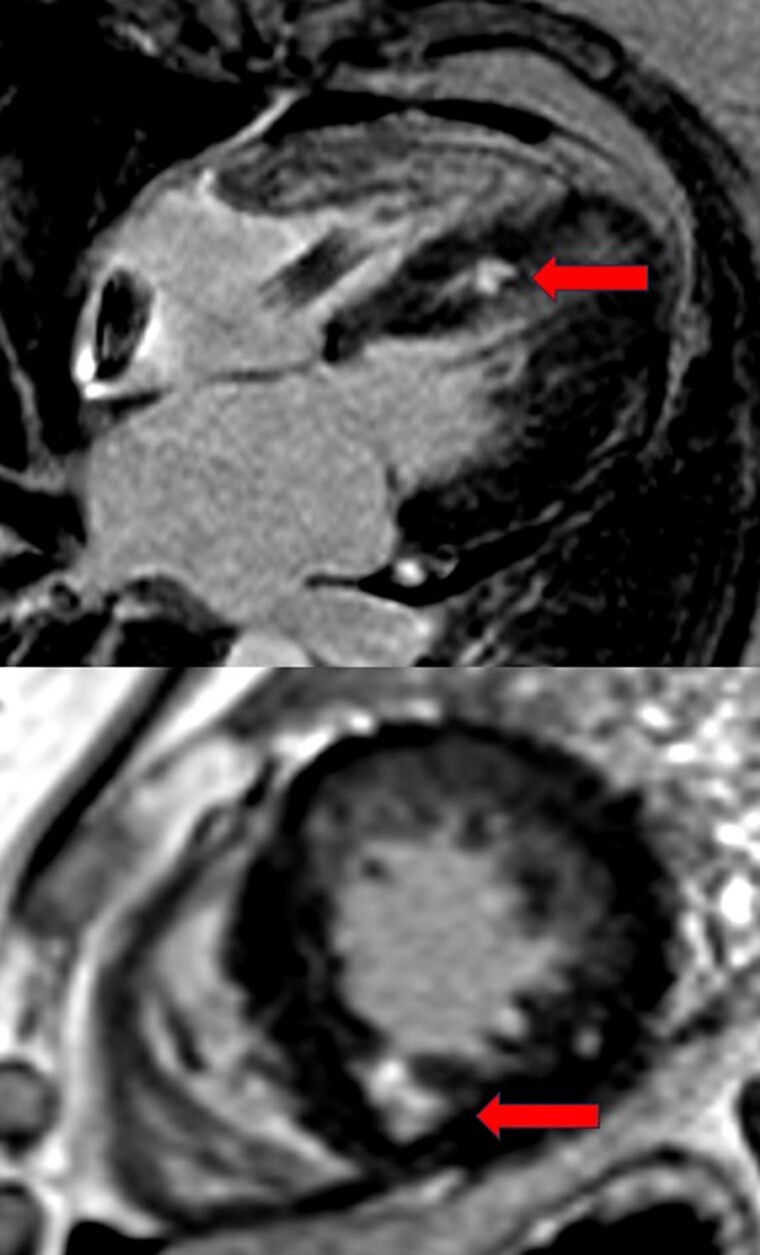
Post-contrast cardiac magnetic resonance imaging displaying subendocardial late gadolinium enhancement at the mid infero-septal myocardial segments in four-chamber long-axis view (top) and in mid short-axis view (bottom), precisely at the location of the left bundle branch area pacing lead (red arrows).
